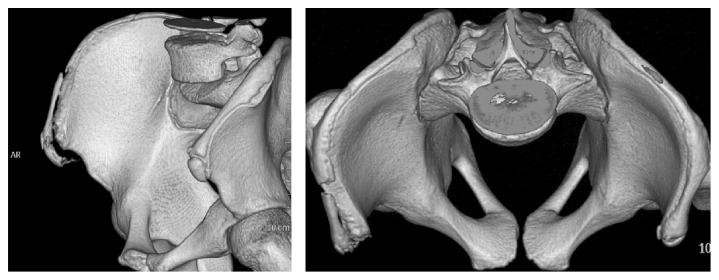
3D CT scan. avulsion of a part of the iliac crest apophysis and the ASIS, downward and more outward.
